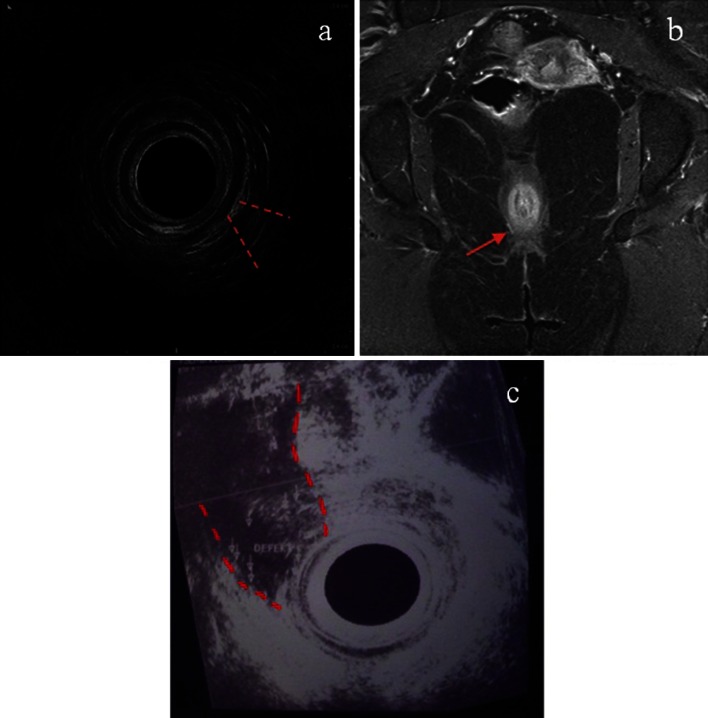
The postoperative anal ultrasound and pelvic magnetic resonance imaging views of patients treated with the hybrid seton (a, b). Note the limited scarring and dehiscence of the sphincter mechanism when the hybrid seton is used, in contrast with the wide defect caused by staged fistulotomy (c), (the sphincter defects are *highlighted* with *red lines* or *arrow*)
